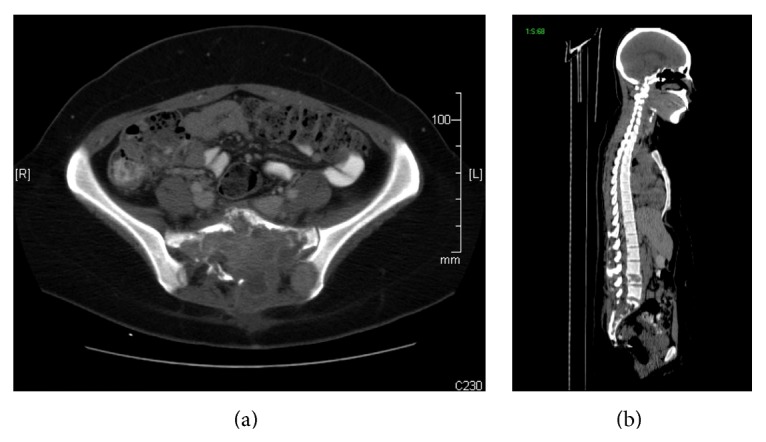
CT Scan showing tumor invasion of sacrum: left and right surrounding parasacral soft tissue (a), and L4 vertebral body (b).
